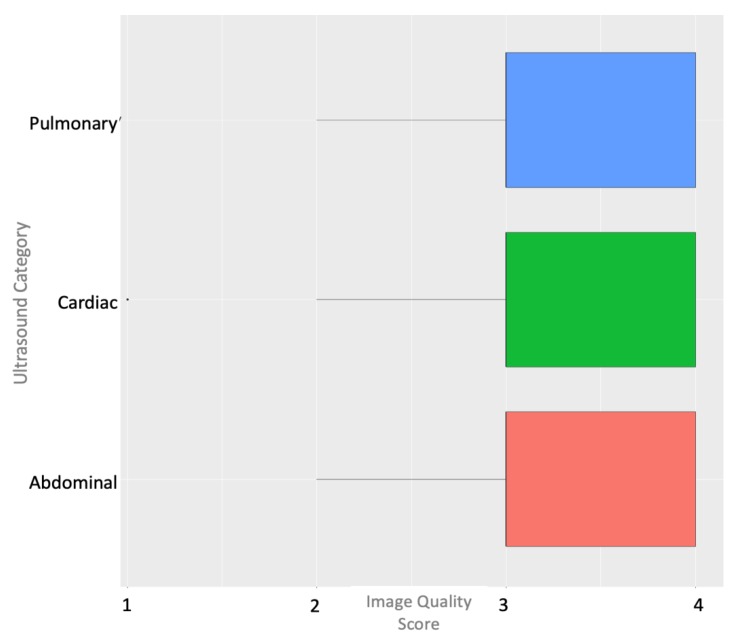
Box plot of image quality scores by ultrasound category.
